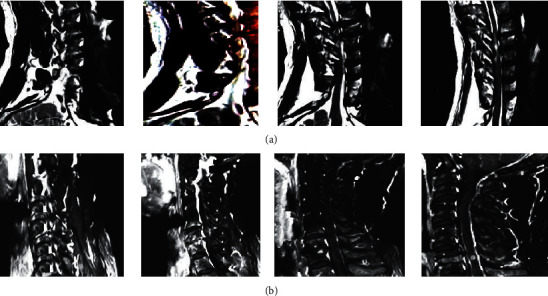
MRI manifestations of a male patient before and after the intervention. The patient was 25 years old and admitted to hospital 3 days after neck and shoulder pain and limb weakness caused by a heavy object hitting the neck. (a) Before the intervention. (b) After the intervention.
